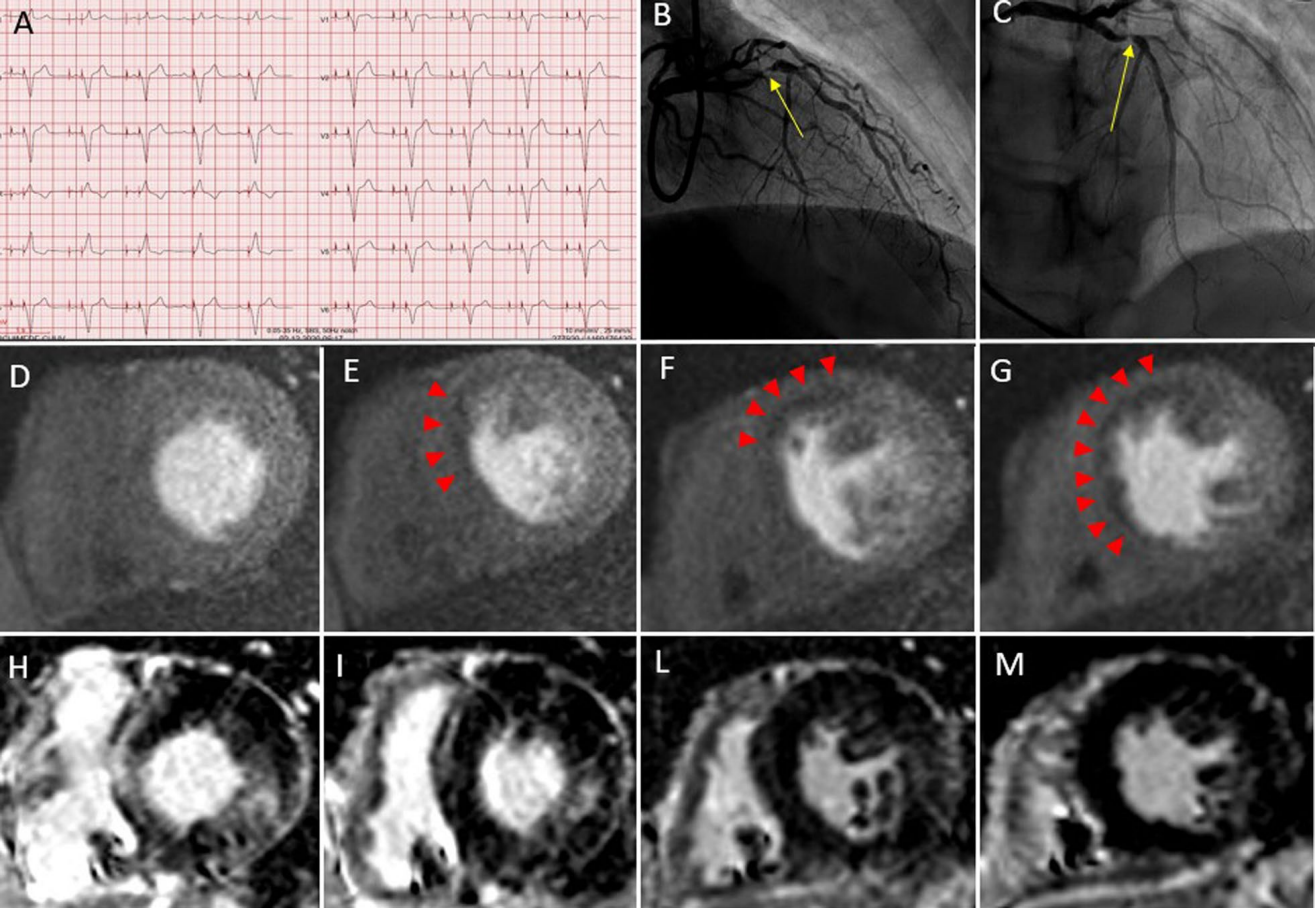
Stress perfusion-CMR in a permanent pacemaker dependent (PPM) patient. A 74-year-old man was referred to stress perfusion CMR due to exertional dyspnea and angor. A double chamber PPM was implanted for a 2-degree atrioventricular block and the patient was PPM-dependent (Panel A). X-ray coronary angiography showed a severe stenosis of the proximal portion of the left anterior descending artery (Panel B, C, yellow arrowed) that was treated by drug-eluting stent implantation. Stress perfusion CMR, performed before x.ray coronary angiography showed an extensive hypoperfusion in the anterior and septal wall (Panel D–G, red arrowed) without the presence of myocardial scar in late gadolinium (LGE) images (Panel H, I, L, M) indicating a positive stress test, consistent with the lesion found in x-ray coronary angiography
